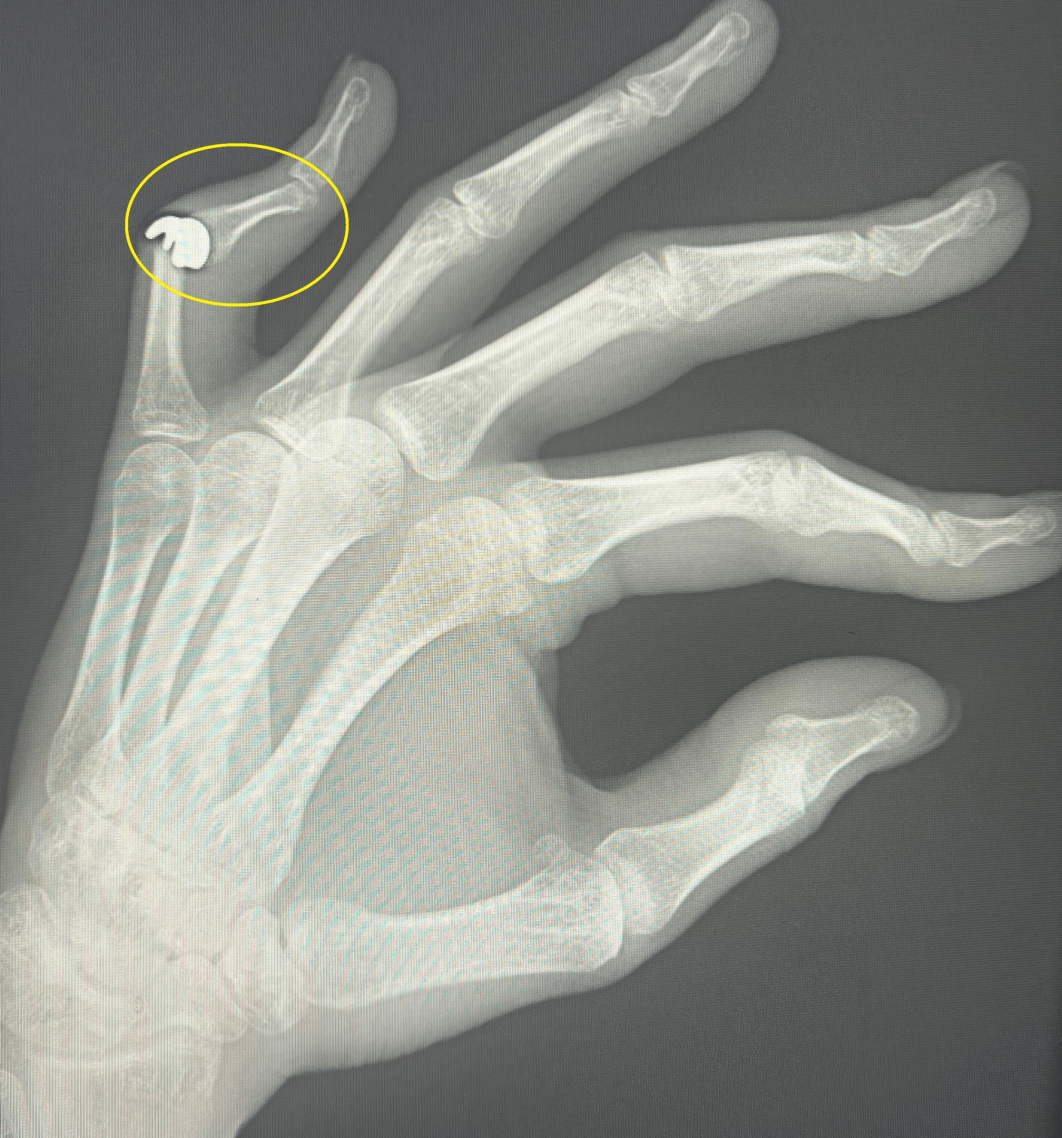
Control X-ray six months after implantation of the CapFlex cementless hemiprosthesis in Robert's projection
